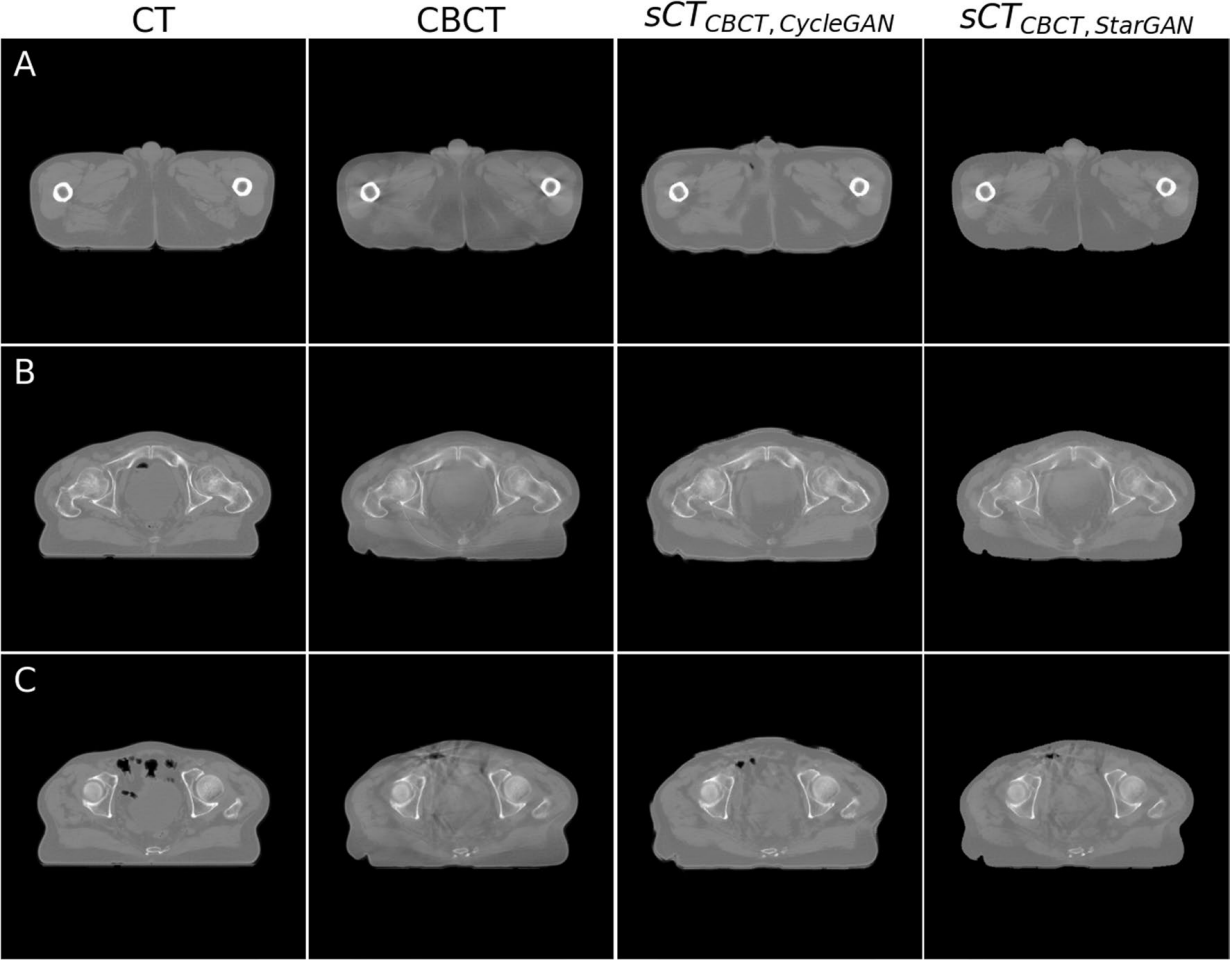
Examples of sCT generated from CBCT using CycleGAN and StarGAN in the testing dataset. A sCT reduced streak artifacts from bone in CBCT, although CycleGAN introduced unreal air regions. B sCT mitigated ring and cupping artifacts in CBCT. C sCT reduced star artifacts originating from air regions in CBCT
